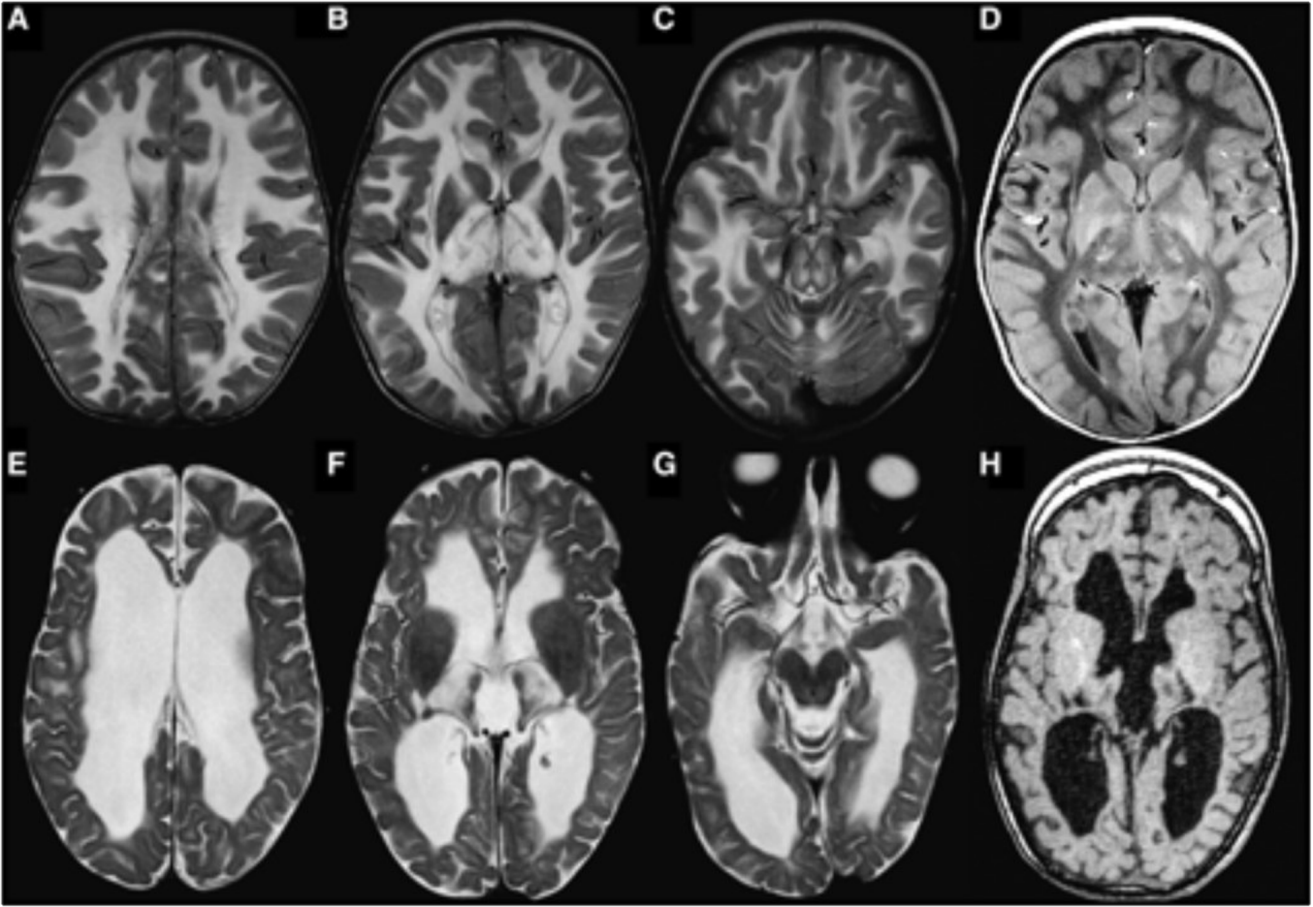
Brain T2-weighted MRI representative of severe LTBL. Axial T_2_- (a–c and e–g) and T_1_-weighted images (d and h) in (EARS2) patient 9 at 8 months (a–d) and 4 years (e–h). Note the diffuse T_2_-hyperintense and T_1_-hypointense signal of the cerebral white matter, only sparing a periventricular rim (a, b, and d). There are also signal abnormalities in the thalami (b) and the midbrain (c). Three years later, there is a serious atrophy of the cerebral white matter and thalami (d, e, and h). The midbrain signal abnormalities have disappeared (f). Reprinted with permission [45].
